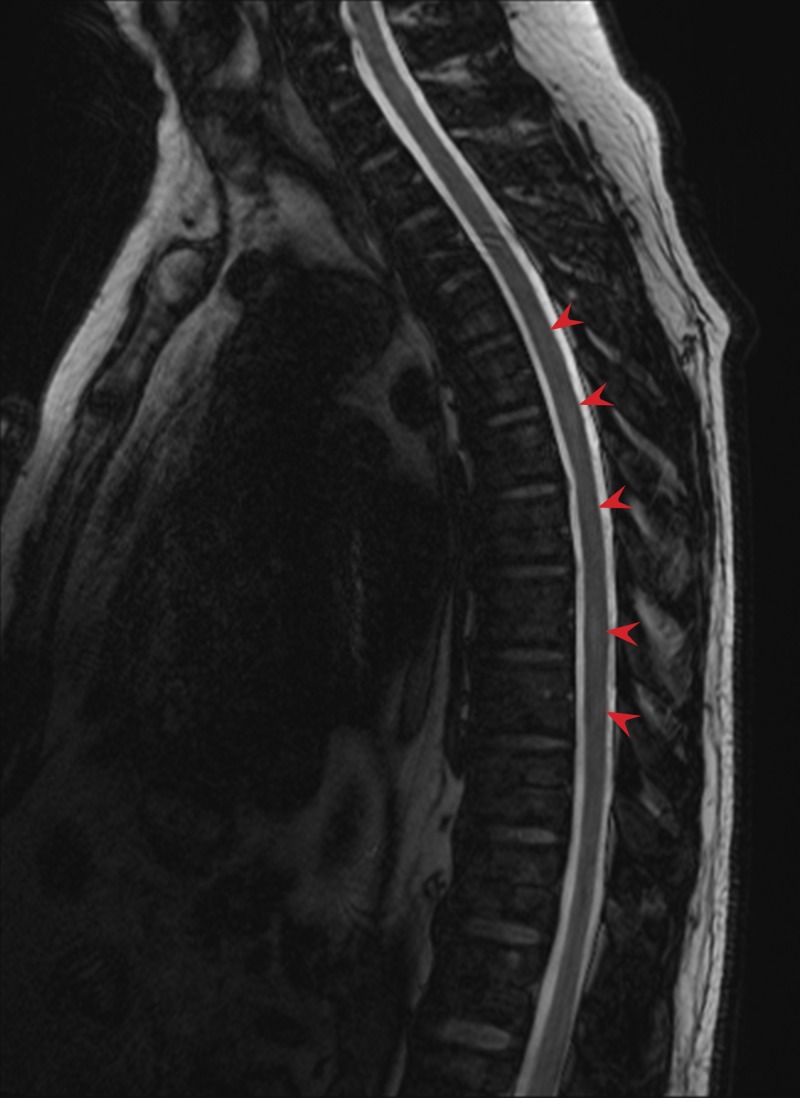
Indeterminate signal abnormality at multiple levels (arrows) of the thoracic and lumbar spine on T2-weighted, Dixon water-fat opposed MRI.
